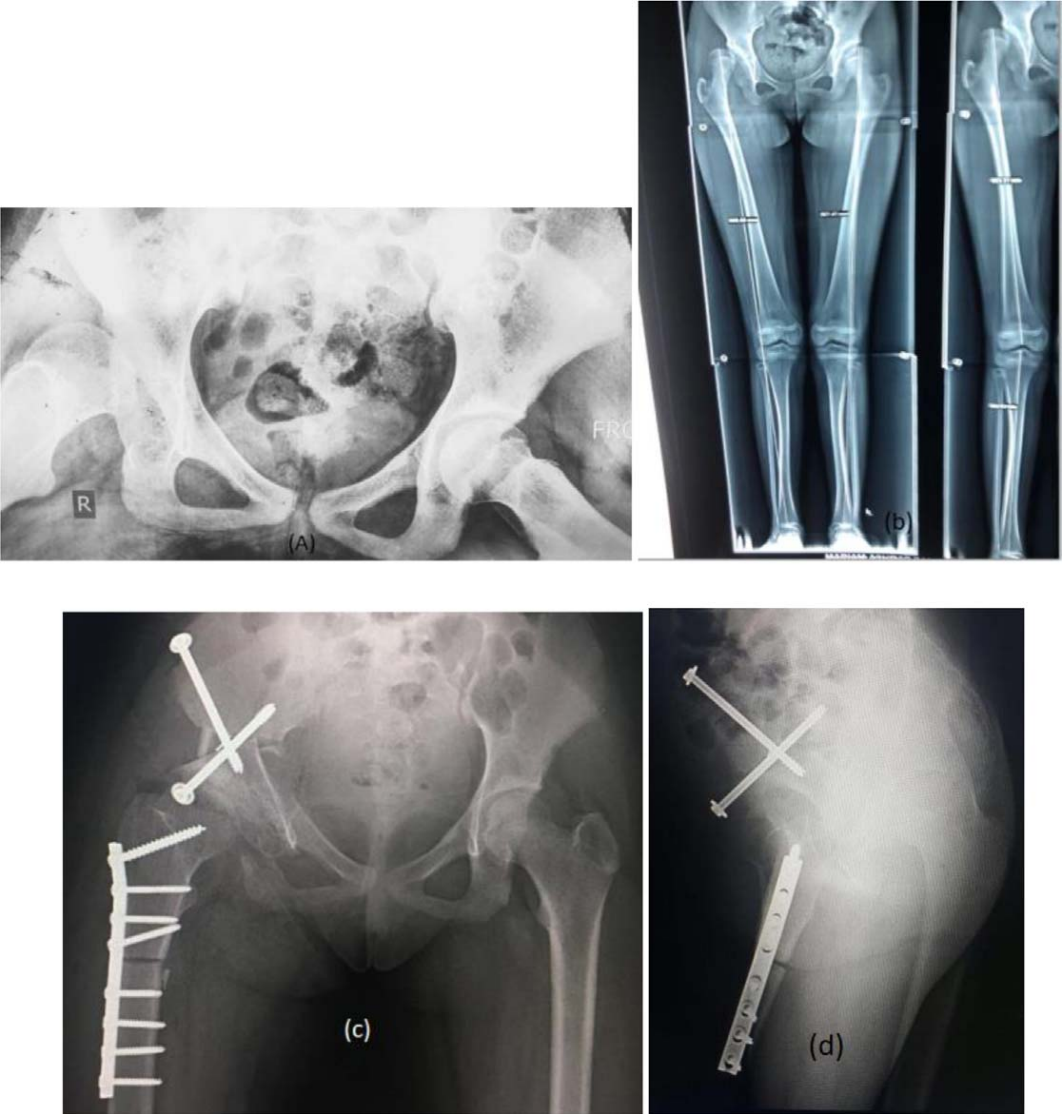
(a) PXR frog lateral demonstrates right hip dysplasia in 13-year-old female. (b) Standing long film demonstrates limb length discrepancy secondary to RT hip subluxation. (c, d). Immediate post-operative plain X-ray AP and lateral of TPO and femoral shortening.
